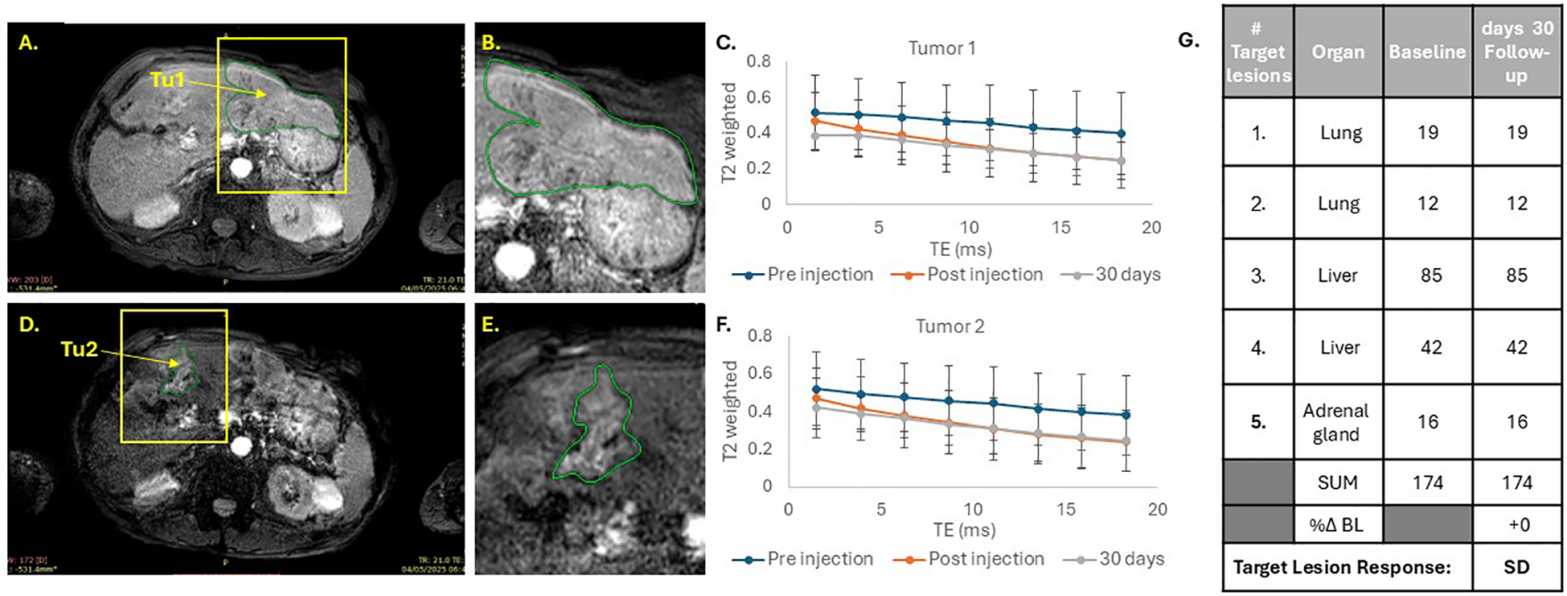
MRI analysis of SaNP accumulation in tumors of patient 1. T2*-weighted MR images (A, B, D, E). Yellow arrows indicate tumor locations. Tumors are marked in green. T2*-weighed signals of tumor Tu1 (C) and tumor Tu2 (F). Blue line – pre-injection (baseline); Orange line – post-injection (~4hrs.) and grey line – 30 days after treatment. Echo time (TE) was measured in milliseconds (ms). CT assessments and RECIST 1.1 evaluation (G).
